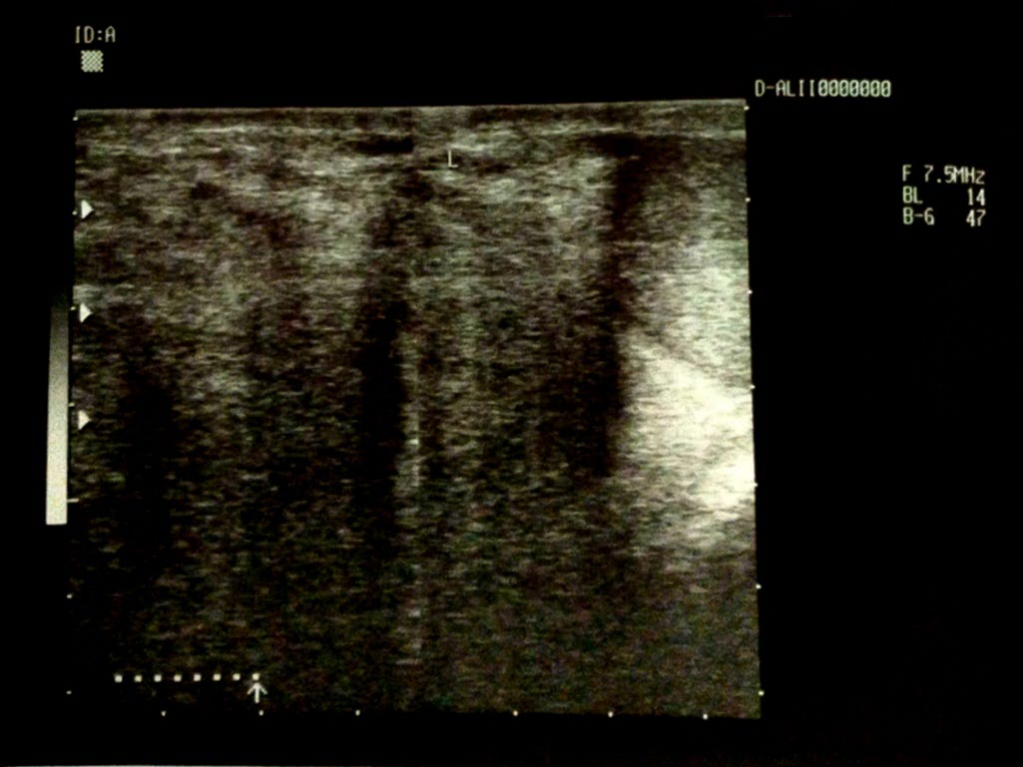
Ultrasonography shows a heterogeneous echogenic area extending from the left inguinal region to the left part of the scrotum.
